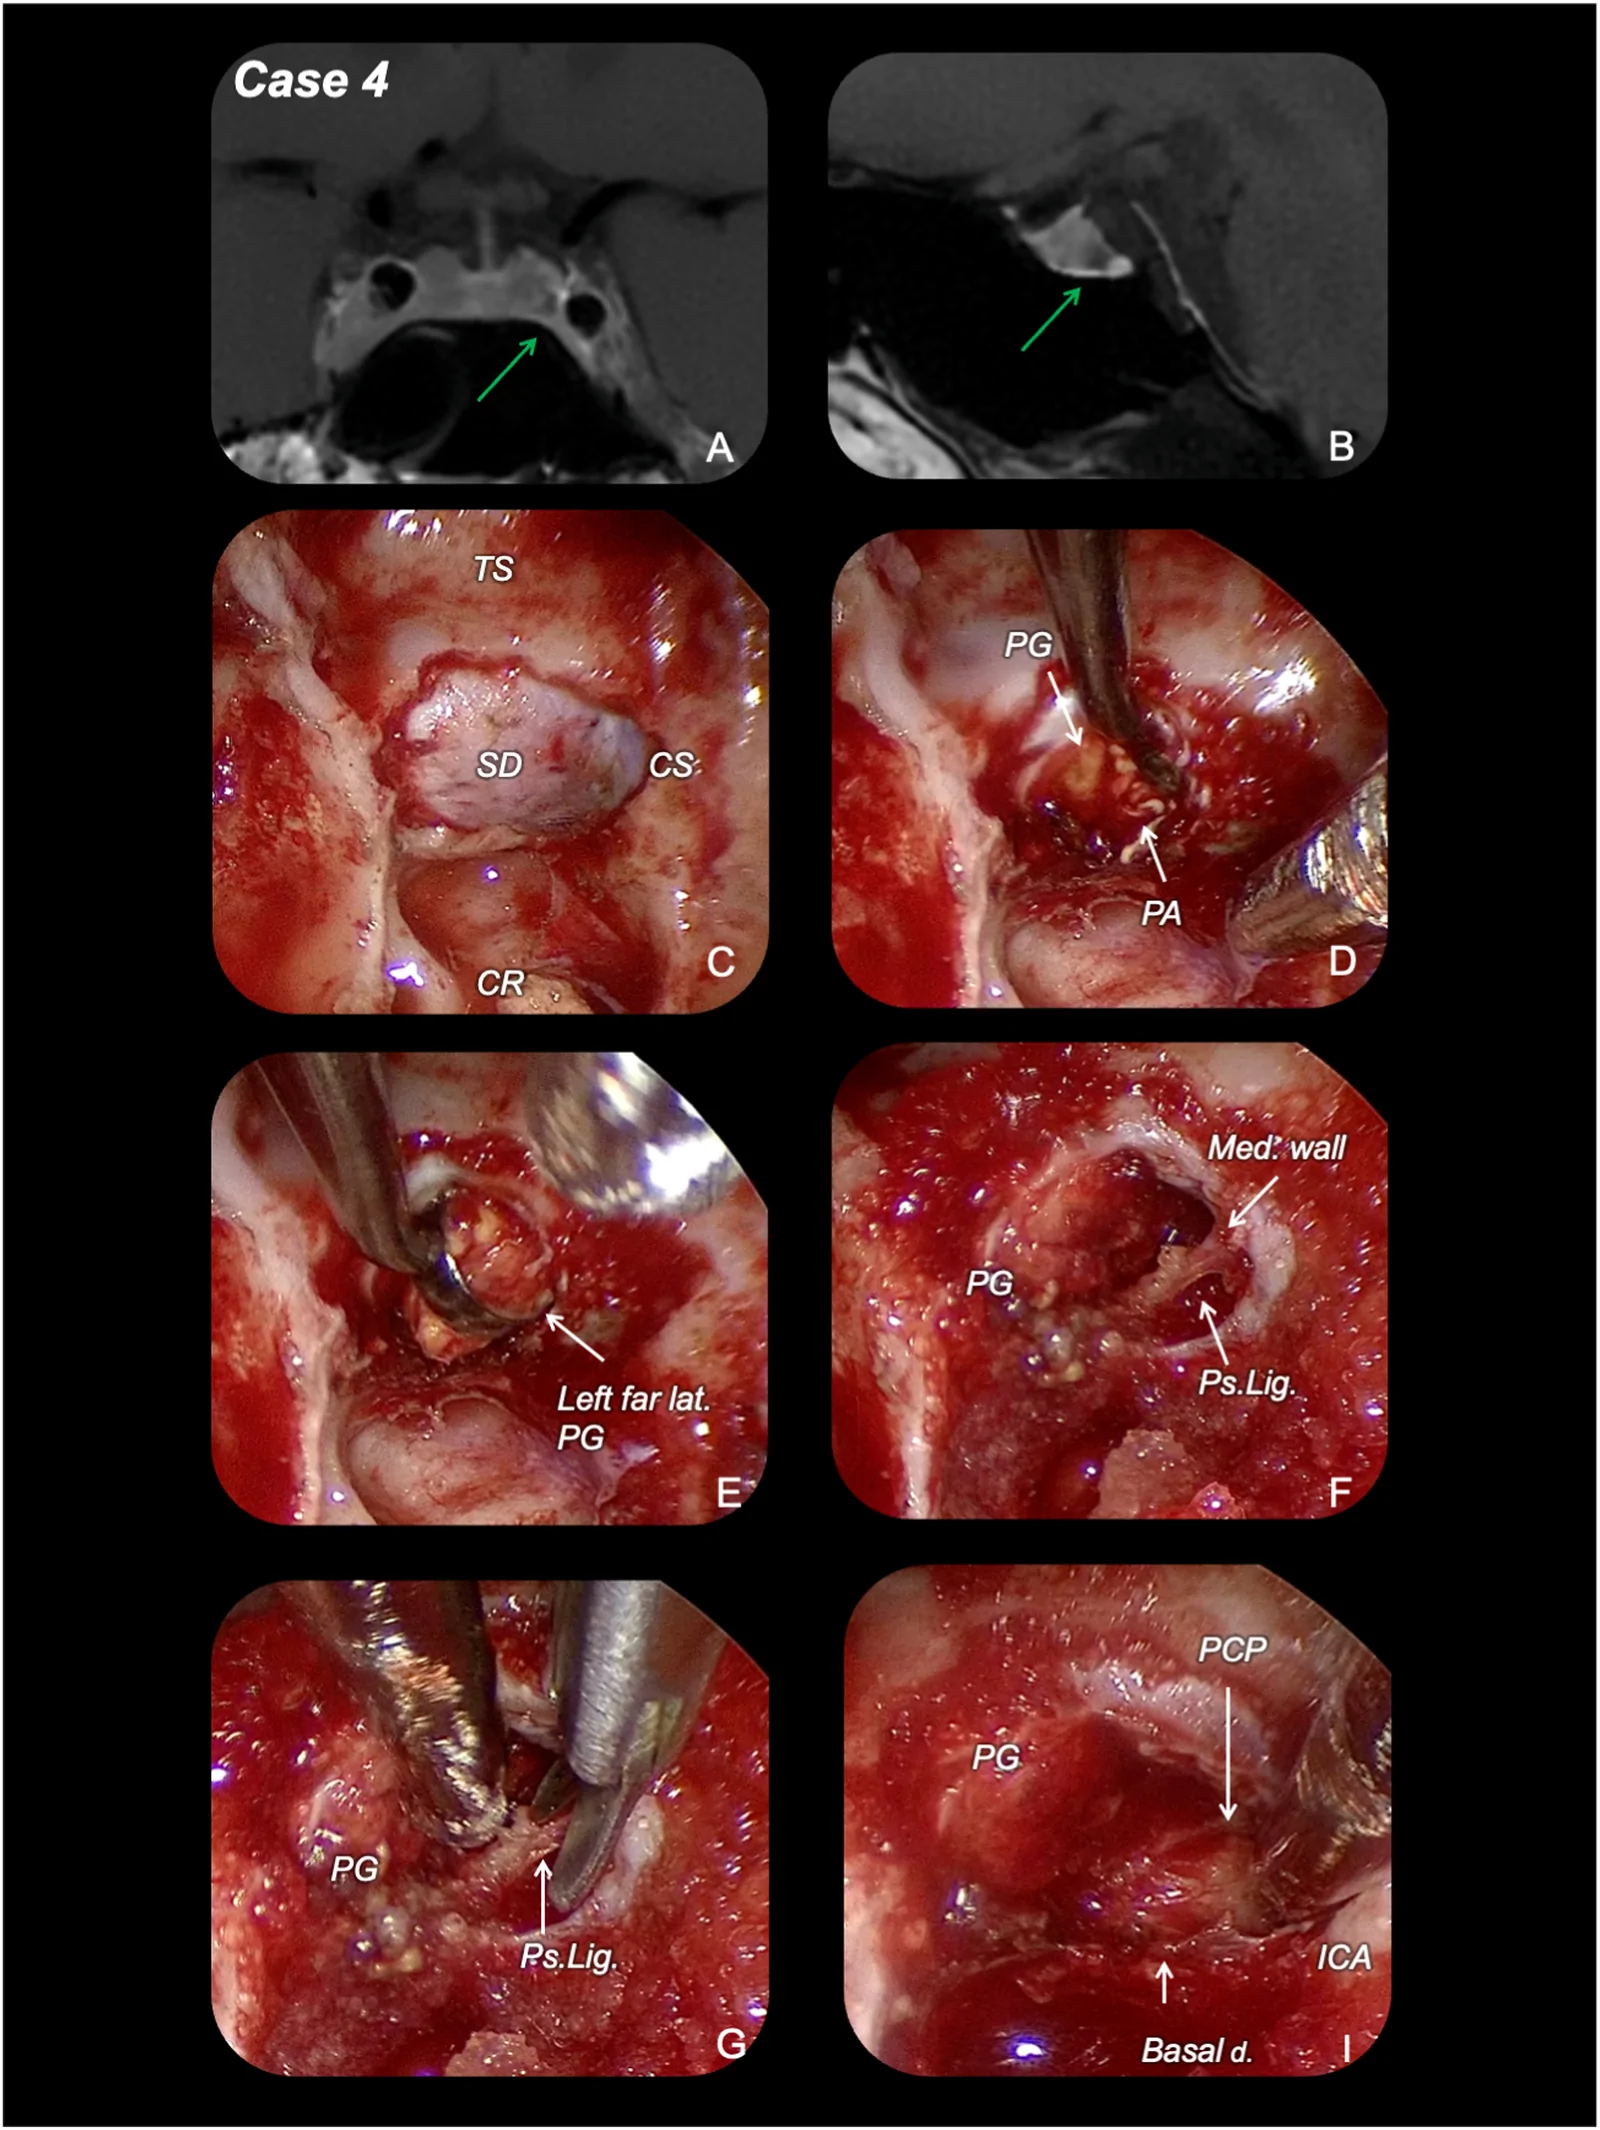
MRI-positive with a microadenoma and cavernous sinus invasion (Case 4) (A–B) Preoperative coronal (A) and sagittal (B) T1-weighted MRI showing a 4-mm left-sided pituitary adenoma (green arrows) with suspected involvement of the cavernous sinus. (C) Endoscopic view after sellar opening showing the sellar dura (SD), tuberculum sellae (TS), cavernous sinus (CS), and clival recess (CR). (D-E) Identification of the pituitary gland (PG) and pathological whitish adenomatous tissue (PA) at the interface between anterior and posterior gland; resection of the far lateral part of the left PG. (F-G) Intraoperative identification of medial wall of the cavernous sinus (Med. wall) invasion and exposure of the parasellar ligament (Ps. Lig.), followed by progressive resection. (G–I) Final aspect of the interdural space between anterior and medial wall of the CS after resection of the PA, showing the surgical cavity, preserved pituitary gland (PG), and exposure of posterior clinoid process (PCP), the internal carotid artery (ICA) and basal dura (Basal d.), with no macroscopic residual tumor.
